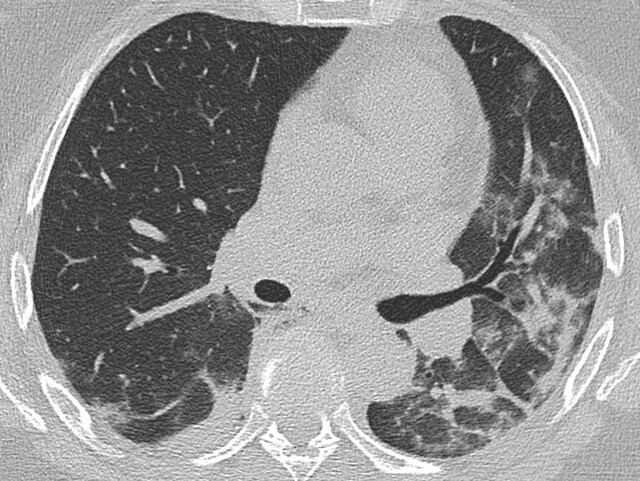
CT at day 9: increasing size and density of previous lesions, onset of new irregular consolidations with parenchymal bands and architectural distortion.
